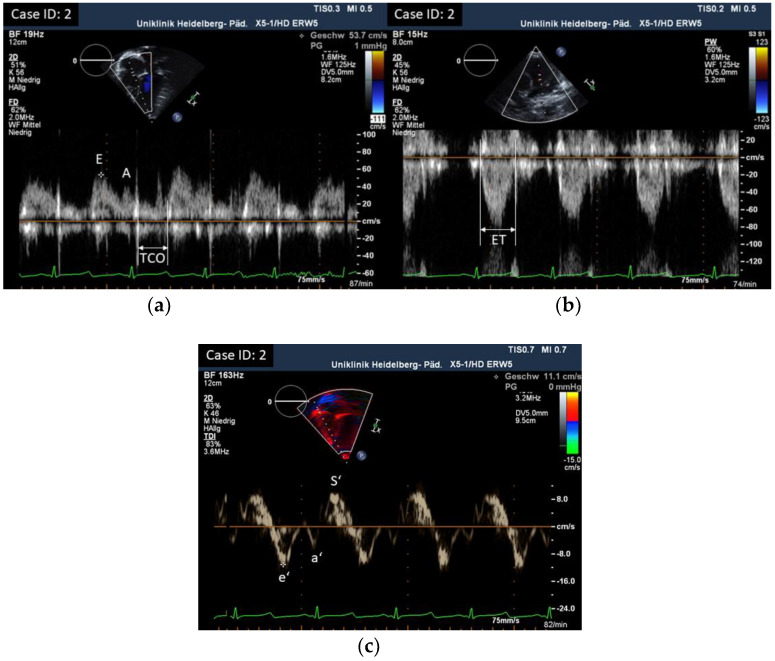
(a) TV E/A inflow and TCO and (b) Pulmonary valve (PV) Doppler and ET by conventional Doppler; RV-MPI = TCO − ET/ET. (c) Tissue Doppler imaging (TDI): TV e’, a’, and S’.
